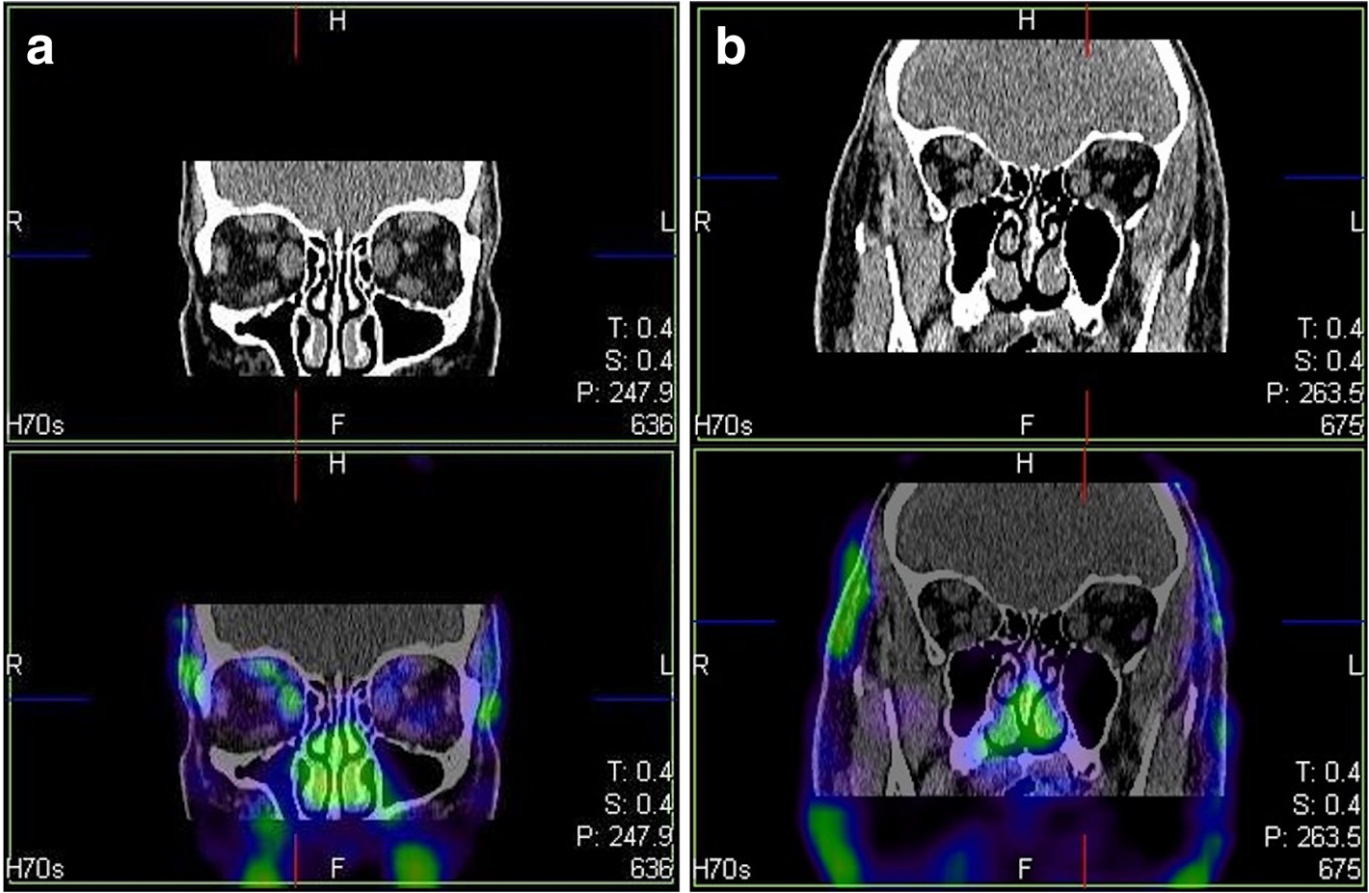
^99m^Tc-DTPA SPET/CT scintigraphy of the orbital cavity in coronal planes. All the rectus muscles of the eye are thickened: a) with increased accumulation of ^99m^Tc-DTPA only in the medial and superior muscles of the right eye, which is indicative of active GO in the medial and superior muscles. b without increased accumulation of ^99m^Tc-DTPA, which is indicative of non-active GO. The physiological 99mTc-DTPA uptake of nasal mucosa is visible at both cases. Abbreviations: MRI = magnetic resonance imaging; SPECT-CT = fused single photon emission computed tomography with computed tomography; GO = Graves’ orbitopathy; ^99m^Tc-DTPA = diethylenetriaminepentaacetic acid tagged with ^99m^Tc
